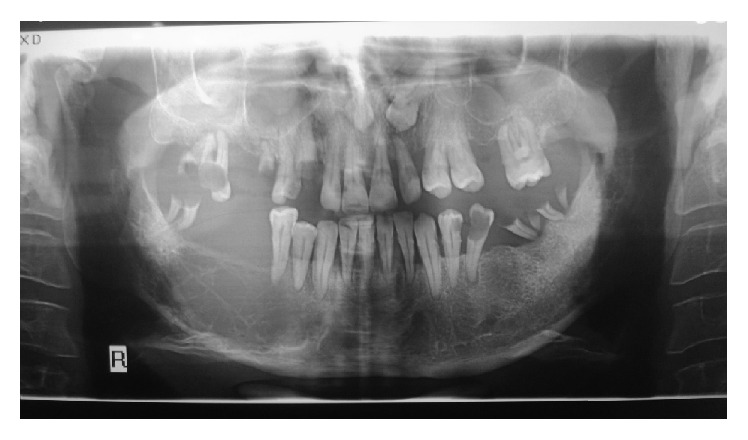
Panoramic radiograph demonstrating a large multilocular radiolucent lesion of the right mandible.
